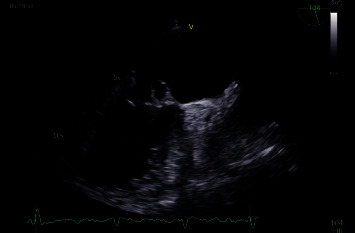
Echocardiogram shows anterior mitral valve leaflet aneurysm.
